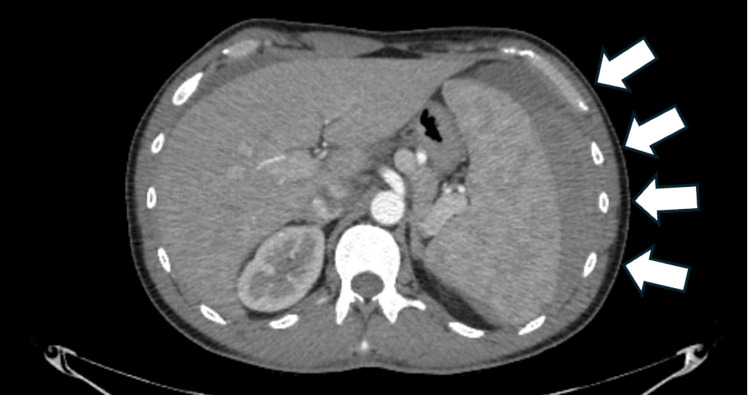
Abdominal CT scan showing splenomegaly, subcapsular hematoma, and hemoperitoneum
